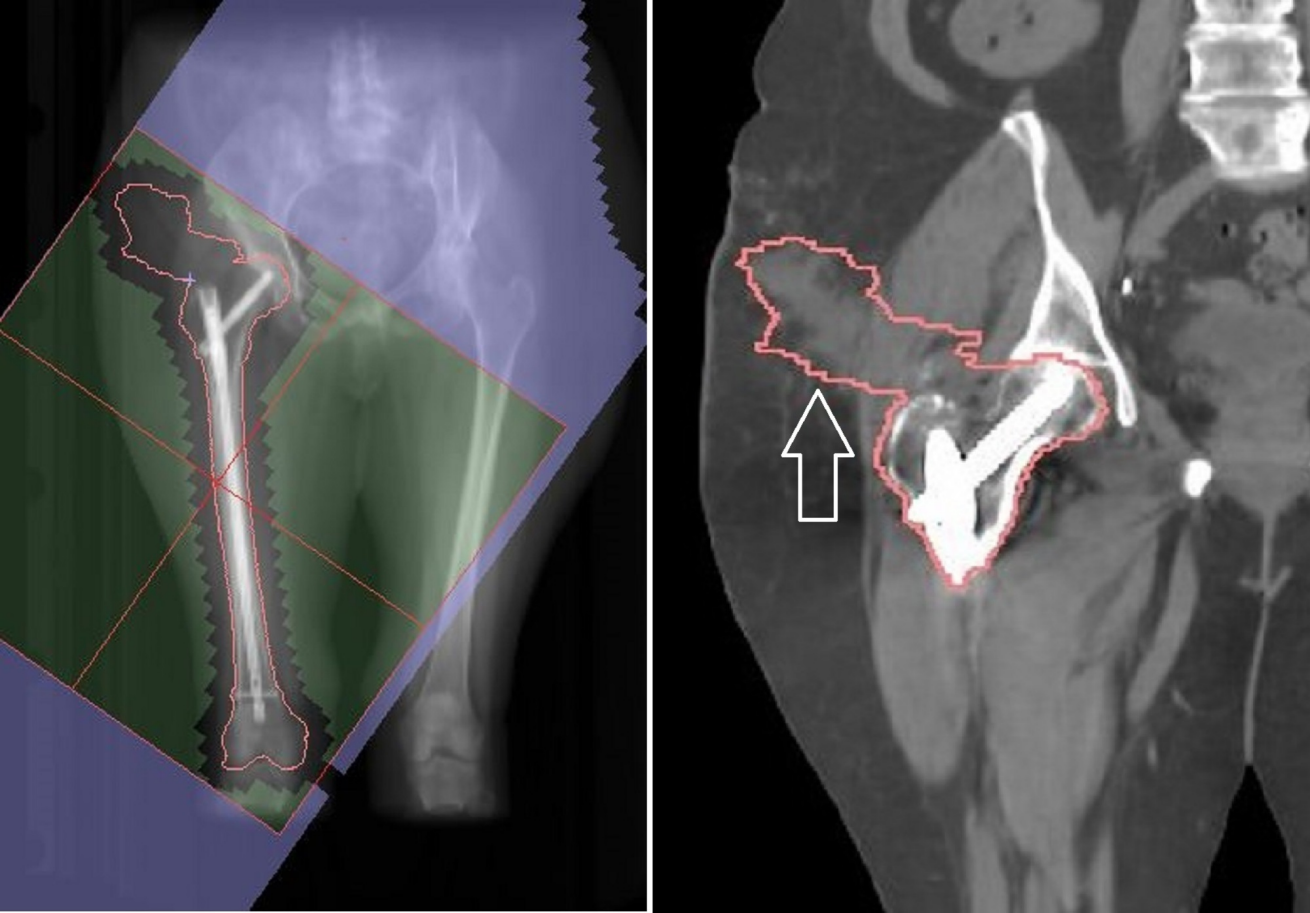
Digitally reconstructed radiograph (DRR) showing suggested volume and typical field (outlined in green) for post-operative radiotherapy after surgical intervention to the femur for bone metastases. Note clinical target volume (CTV) extending along surgical scar tract (white arrow) and treatment of entire prosthetic material.
